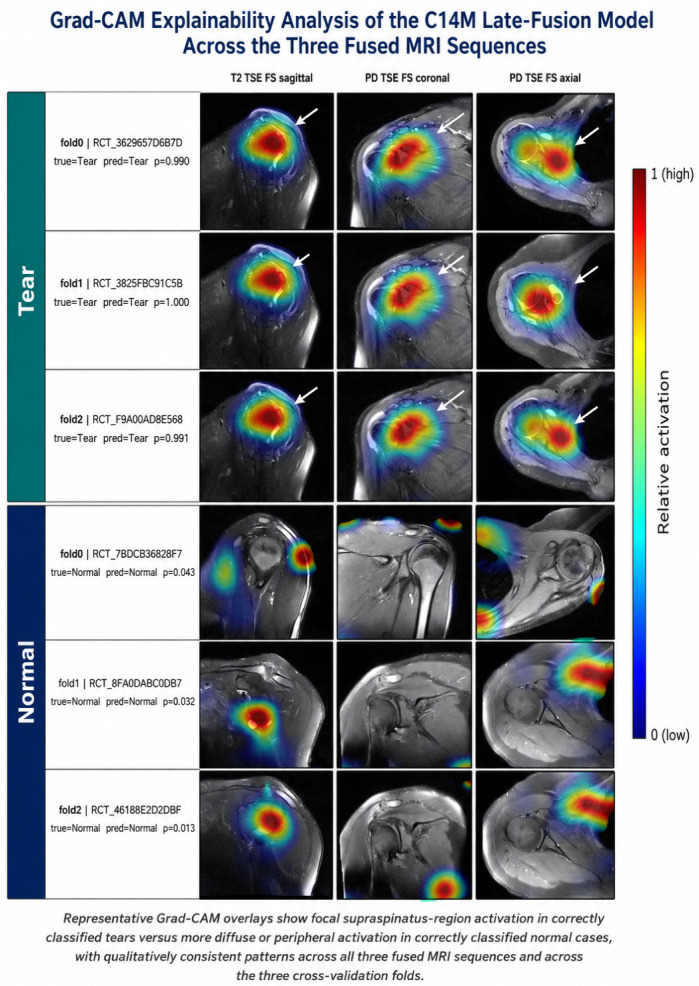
Out-of-fold Grad-CAM explainability matrix for the task-optimized late-fusion model (C14M) across the three fused MRI sequences (T2 sagittal, PD coronal, and PD axial). Rows are stratified by true patient pathology (Tear vs. Normal) across independent validation splits (folds 0, 1, and 2), with anonymized patient identifiers and true/predicted classes indicated. White arrows highlight the localized, highly focused supraspinatus-region activation in true tear cases, contrasting with the diffuse or peripheral background activation patterns observed in true normal/negative controls. The color bar represents relative feature activation maps normalized from 0 (low) to 1 (high). TSE: turbo spin-echo, FS: fat-suppressed, PD: proton density.
